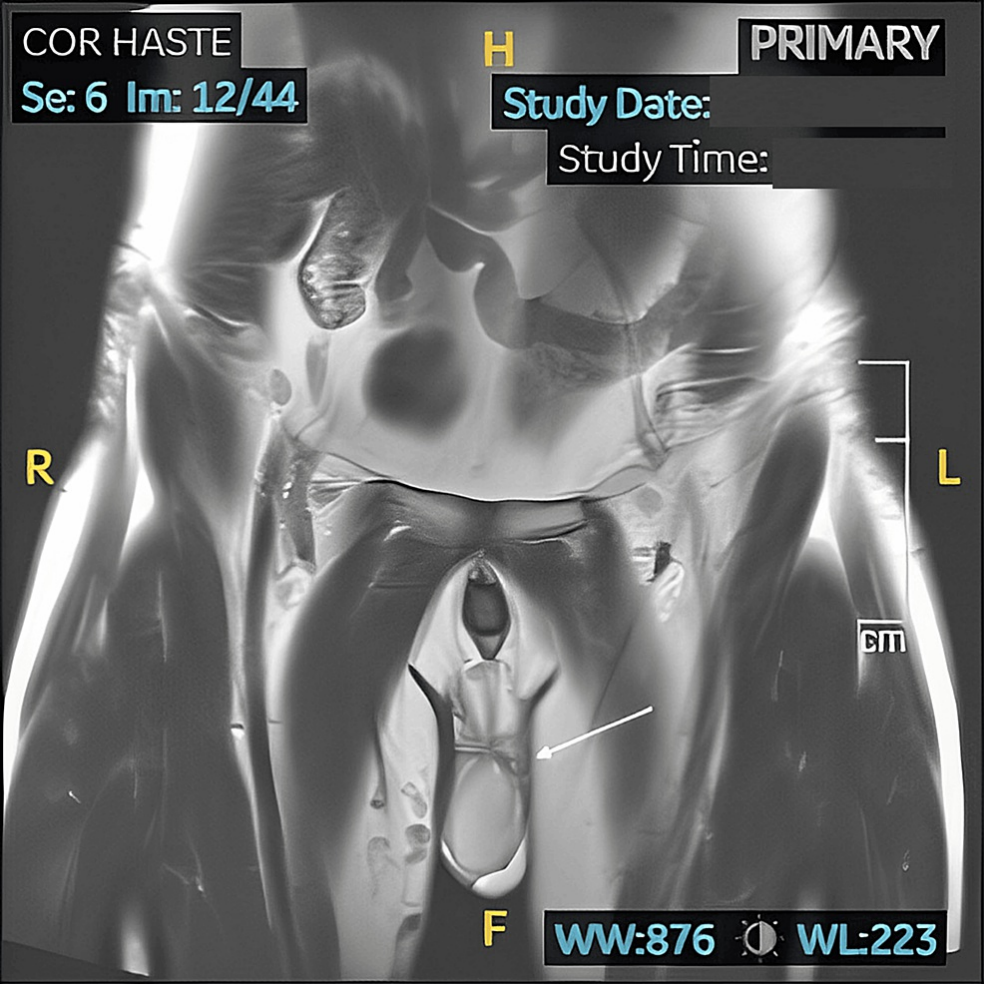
MRI of the pelvis in the coronal plane showing varicosities in the left hemiscrotum
